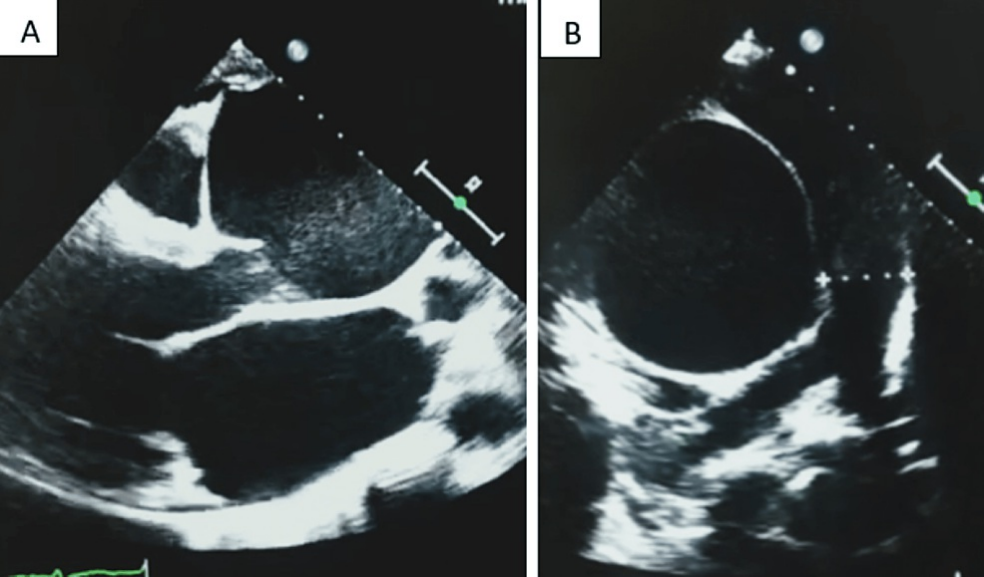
(A) Long-axis view on TTE showing a giant aortic root aneurysm with no evidence of intimal flap or pericardial effusion. (B) Short-axis view visualizing the giant aneurysm. TTE: transthoracic echocardiography.
